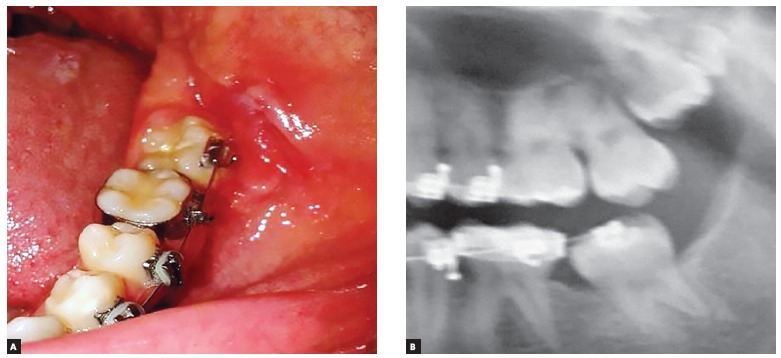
Aspect after 12 months, showing the eruption of tooth #38: A) clinical aspect; B) radiographic image.
